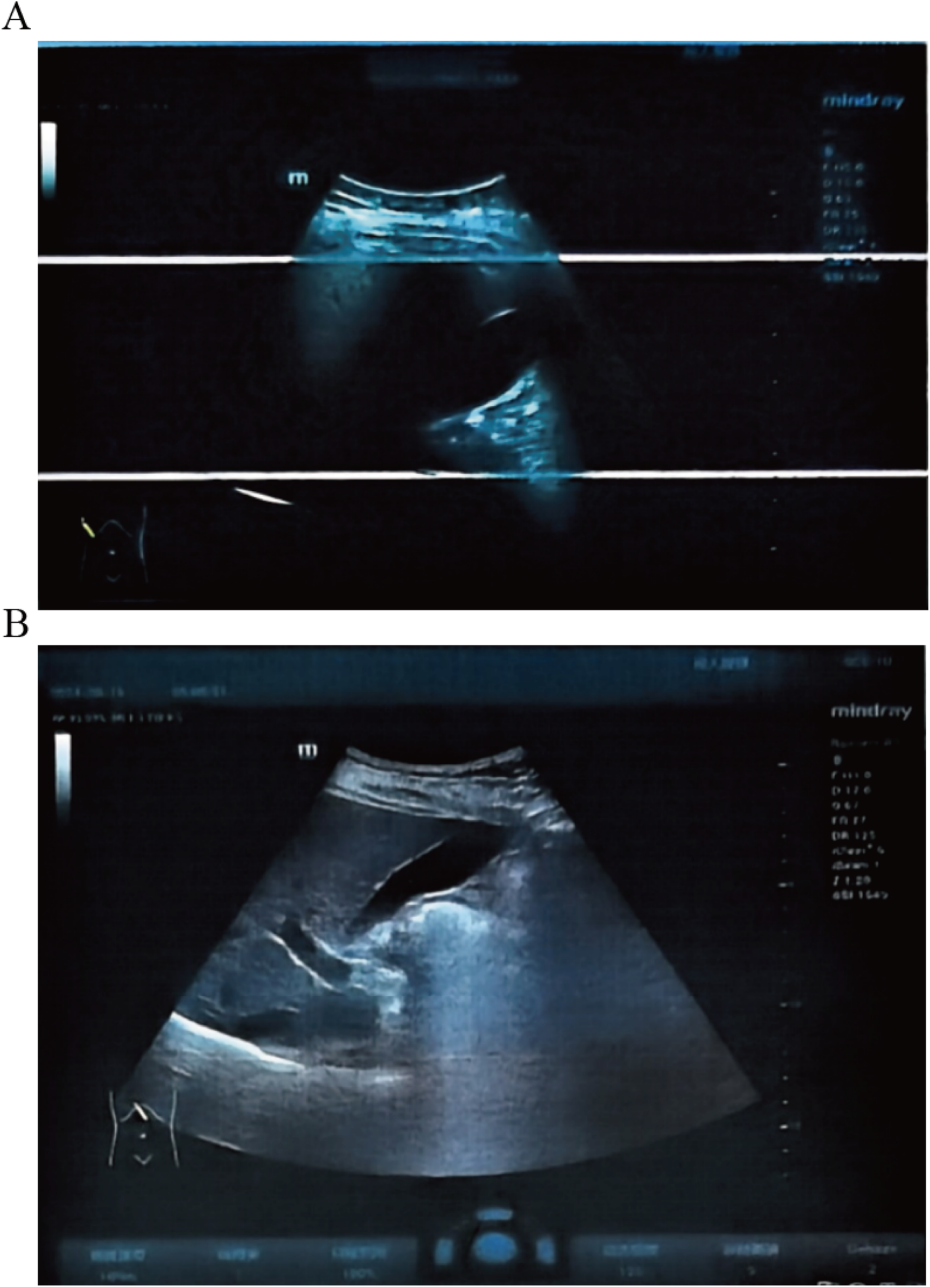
Liver ultrasound before and after Mazdutide treatment. (A) Baseline imaging shows hepatic steatosis with hepatomegaly and increased echogenicity. (B) Week 14 follow-up shows normalized liver size and echotexture, indicating resolution of steatosis.
